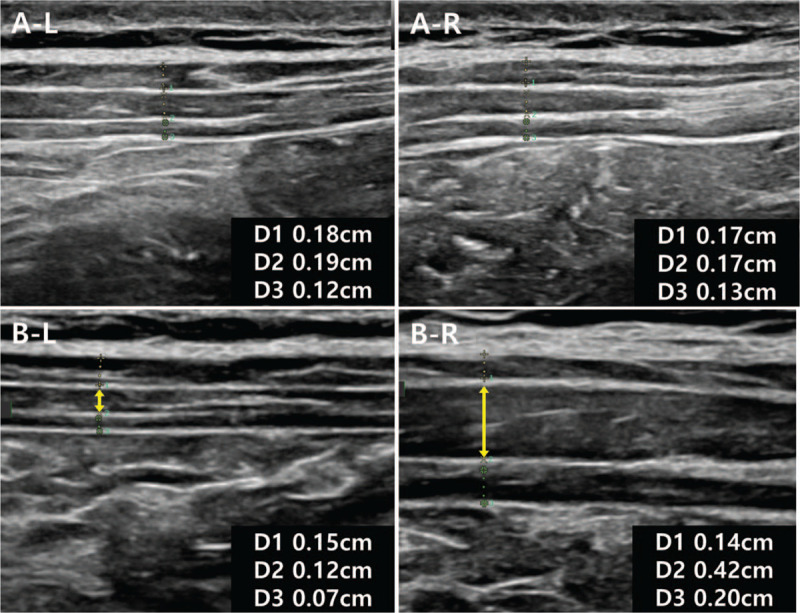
The thicknesses of the 3 abdominal muscle layers, namely the external oblique (D1), internal oblique (D2), and transversus abdominis muscles (D3), were measured using ultrasonography in the supine (A) and standing positions while the patient blew the positive expiratory pressure device constantly at 20% strength of the measured maximal expiratory pressure (B). Each measurement was performed in the area with the most severe herniation (left, L) and on the opposite side (right, R).
